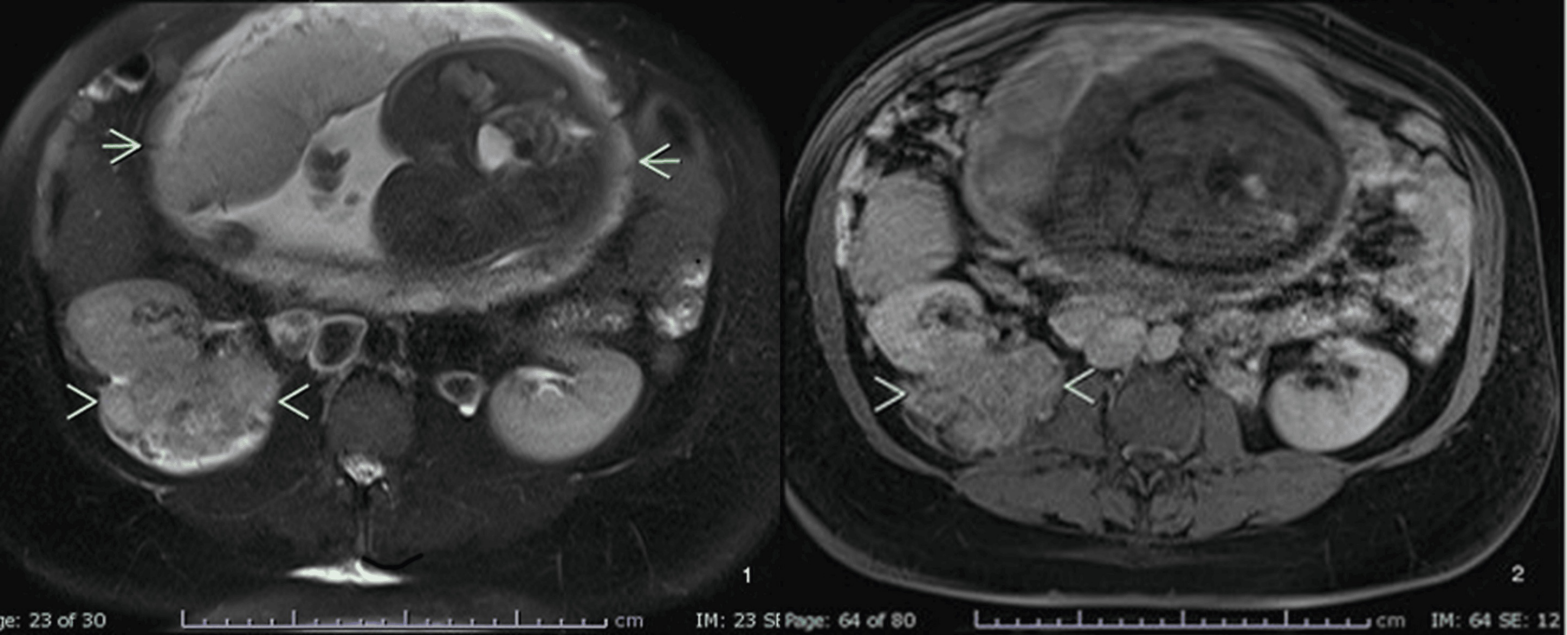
MRI at the third trimester of pregnancy revealed a large heterogeneous right-sided renal mass. On T1- and T2-weighted, MRI images, the mass is marked with arrowheads and the fetus is marked with short arrows.
